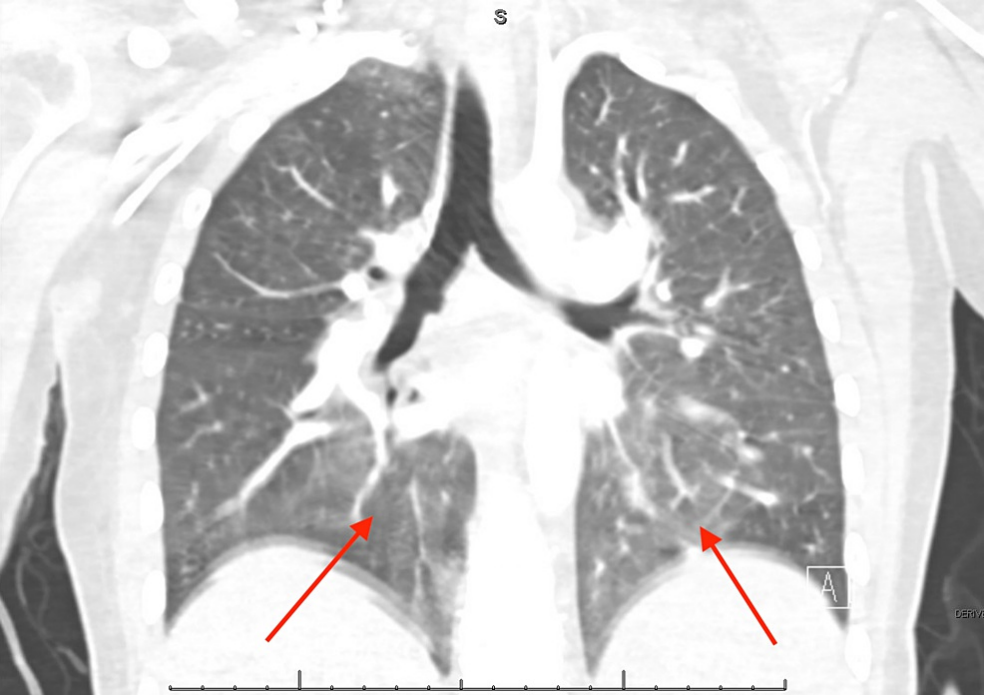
Computed tomography (CT) chest showing patchy ground glass opacities throughout the lower lobes Red arrows show new ground glass opacities
